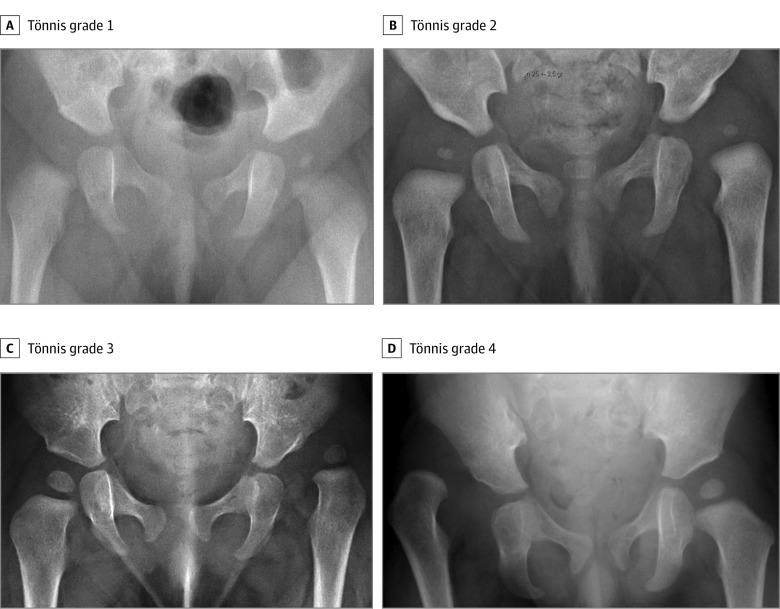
Tönnis Classification A, Grade 1: the center of the femoral head lies medial to the Perkin line (a vertical line drawn from the most lateral point of the acetabulum). Radiograph is of an infant girl with a dislocatable right hip discovered at routine examination. B, Grade 2: the center of the left femoral head lies lateral to the Perkin line. Radiograph is of an infant girl referred because of decreased hip abduction at routine examination. C, Grade 3: the center of the left femoral head lies at the level of the lateral aspect of the acetabulum. Radiograph is of an infant girl referred because of limited abduction. The mother had noted shortening of the leg. D, Grade 4: the center of the right femoral head lies above the lateral aspect of the acetabulum. Radiograph is of a male child referred because of leg length discrepancy. The parents had sought medical attention for some time before the diagnosis was made.
